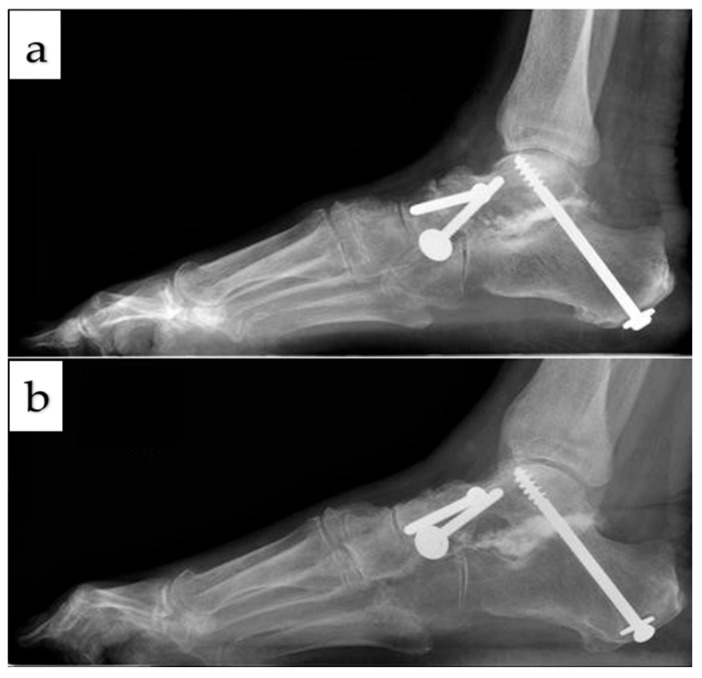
Follow-up radiographs at >12 months (a) and at final follow-up >10 years (b).
